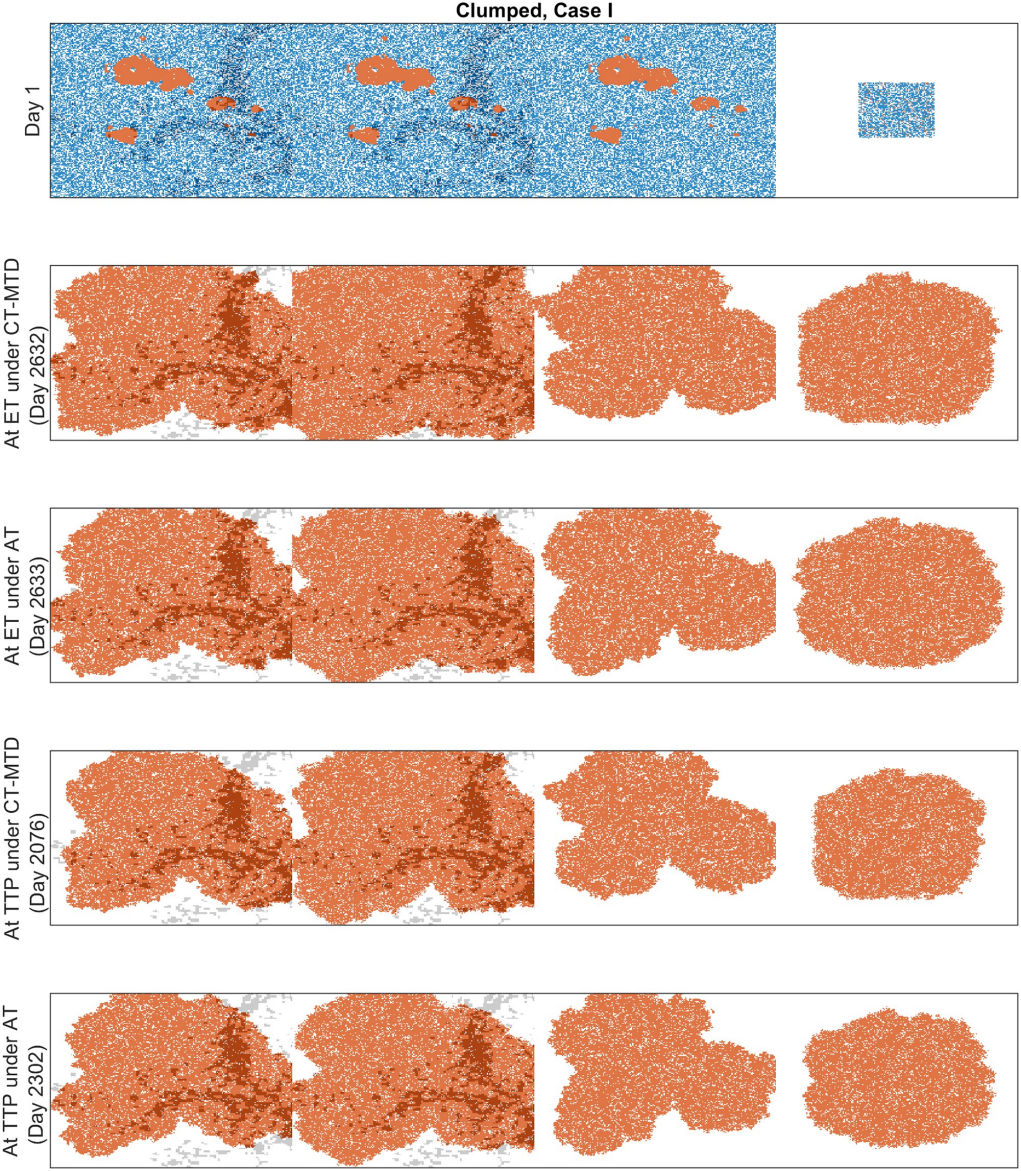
Cell configurations at the ET and TTP under CT-MTD and AT for Case I with the clumped invisible metastasis. The red, blue, and white dots correspond to R-cells, S-cells, and empty sites. The gray dots show the sites that are accompanied by fibroblasts.
